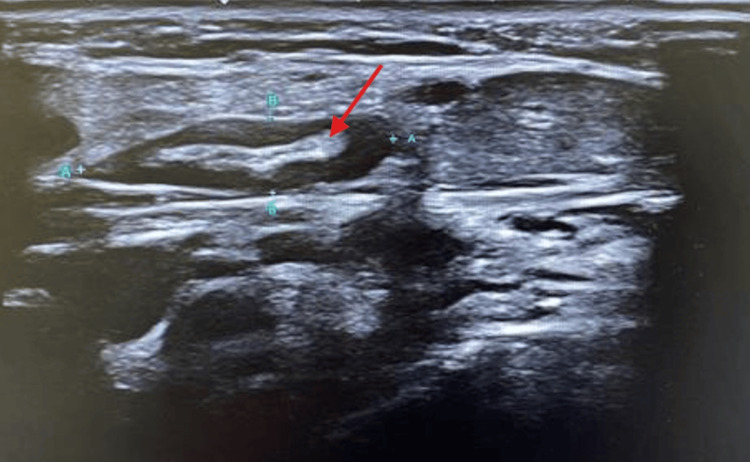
Ultrasound image of the right neck showing an echogenic structure (thrombus, red arrow) within the lumen of the internal jugular vein (delimited by letters A and B, in blue)
